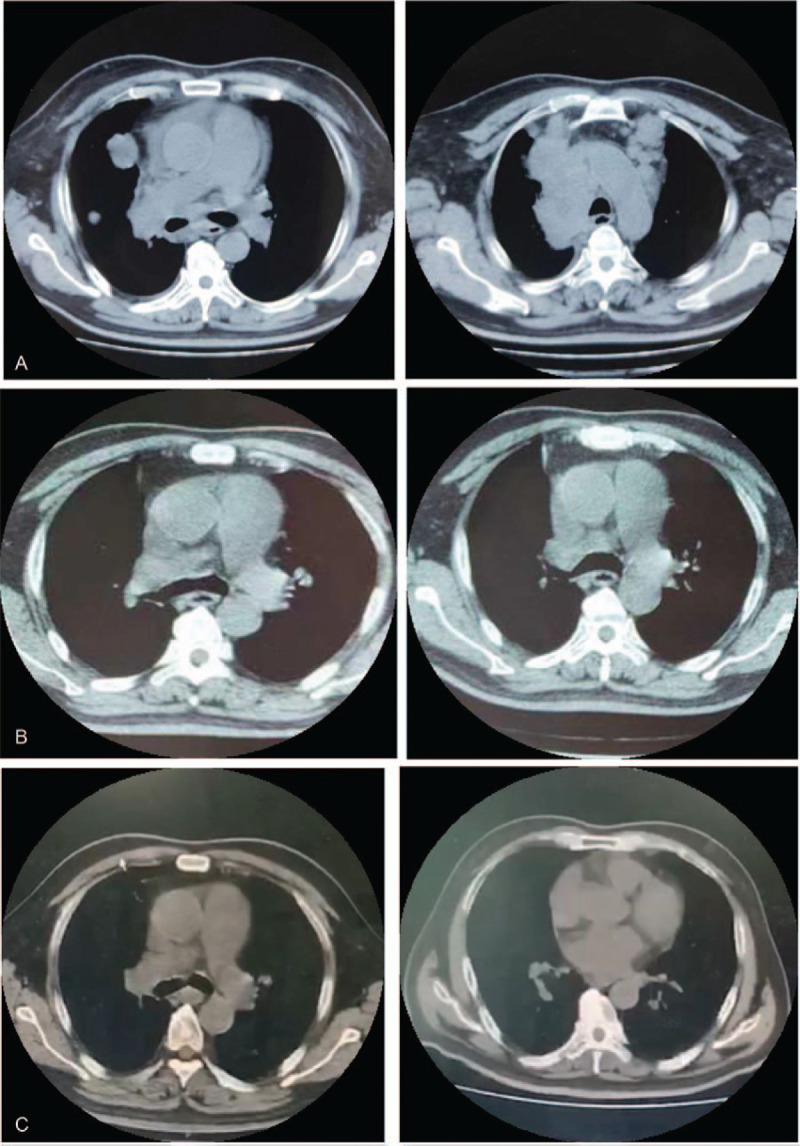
A. CT scan showed right hilar mass with unclear edges extending to the right middle lobe and mediastinum accompanied by a few right pleural effusion. B. Two cycles after treatment (May 2, 2019), therapeutic evaluation was considered as a complete remission (CR) and the size of mass reduced remarkably. C. Four cycles after treatment (June 20, 2019), the efficacy was partial remission (PR).
